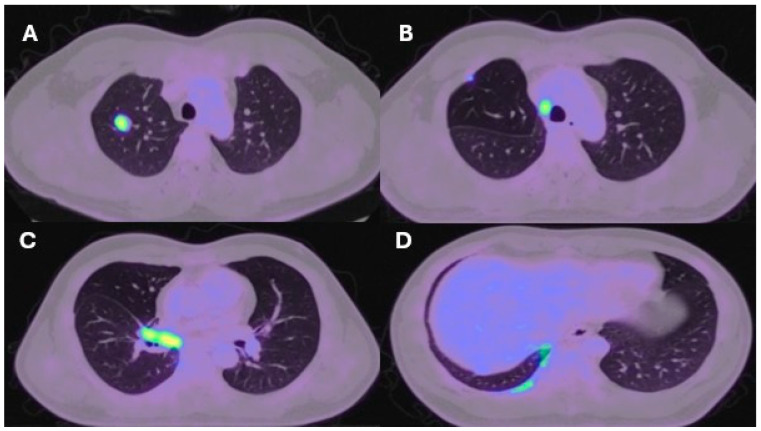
PET-CT axial slice of a right upper lobe stage I lung cancer (A). Post-operative recurrence in the upper paratracheal regions on PET-CT (B). Recurrence at the right hilum (C). Recurrence in the posterior ribs on the right-hand side (D).
